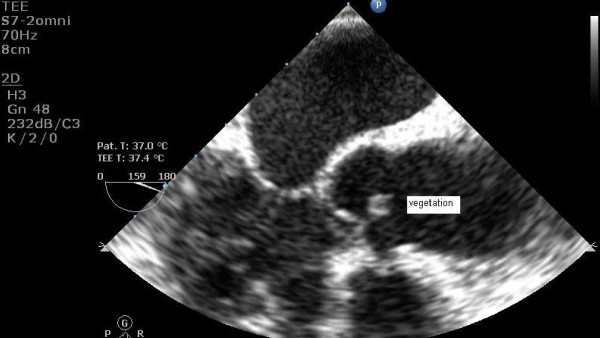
Transthoracic echocardiography, showing a vegetation of about 1 cm on the aortic valve.
